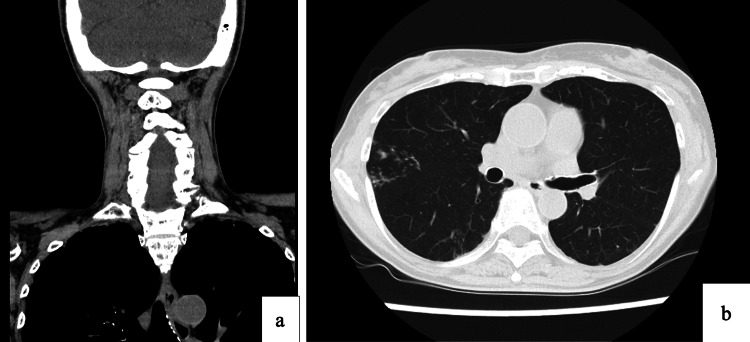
(a, b) CT images on postoperative day 5 confirming the resolution of the gas collections observed immediately post-surgery CT: computed tomography
